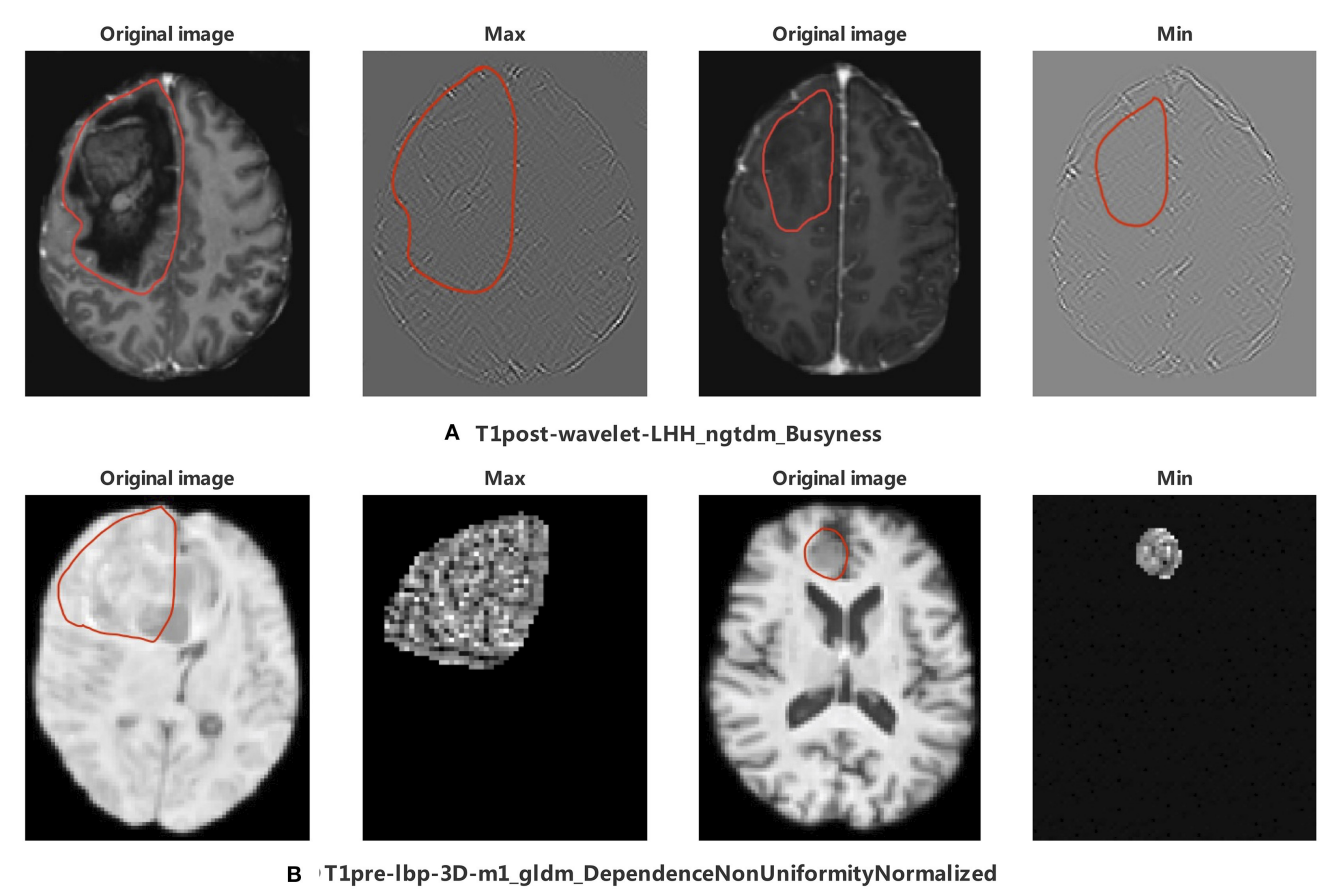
The appearance of the MR image corresponds to top 2 radiomics features in Oligodendroglioma. The region encircled in red represents the legion boundary. (A) wavelet images and corresponding original images of the maximum and minimum value of T1post-wavelet_LHH_ngtdm_Busyness. (B) LBP images and corresponding original images of the maximum and minimum value of T1pre-lbp-3D-m1_gldm_DependenceNonUniformityNormalized. LBP image only included mask region in PyRadiomics (35).
